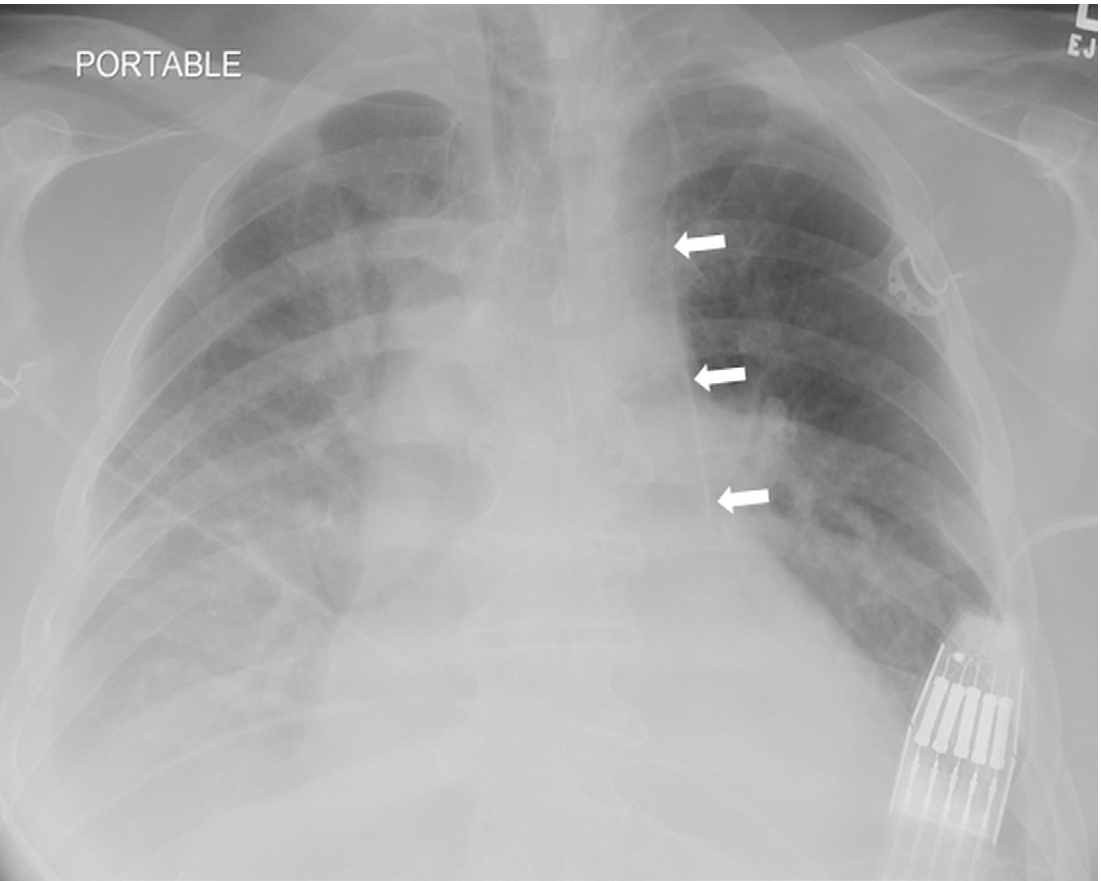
Chest radiograph shows left paramediastinal position of the central venous catheter (arrows) inserted via left internal jugular vein.
